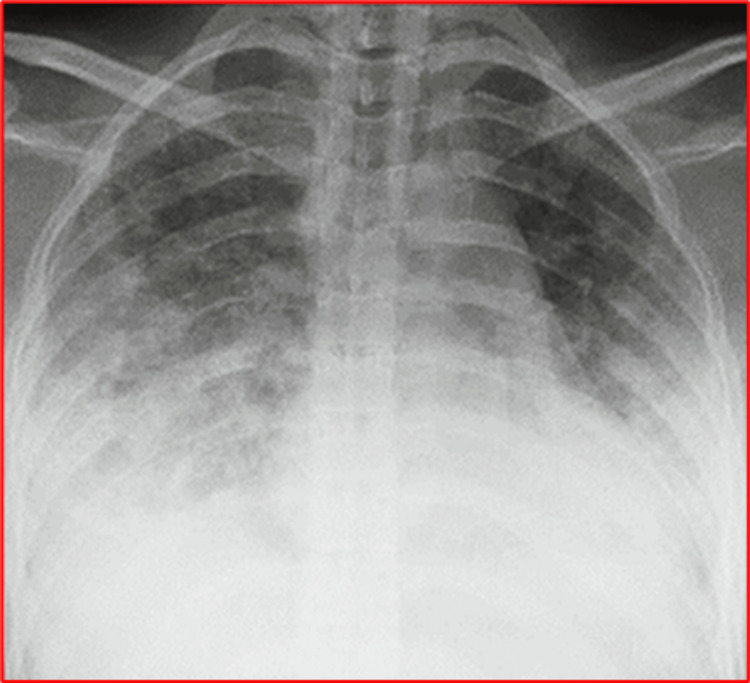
Chest X-ray showing diffuse infiltrates. This figure was obtained from Dr. Nissar Shaikh’s image collection; the patient's informed consent was obtained.
